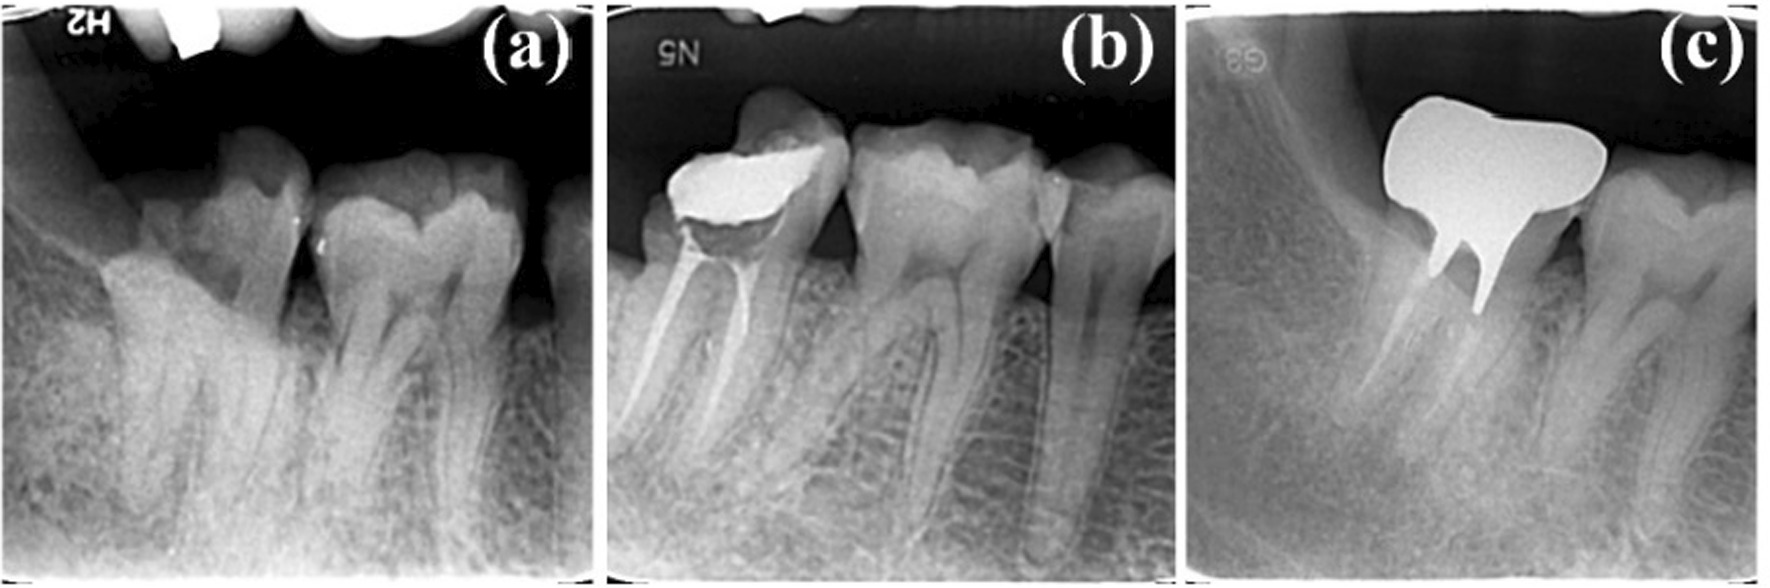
a X-rays at initial diagnosis, b X-rays after root canal treatment, c X-rays of 4 years after the restoration was completed
